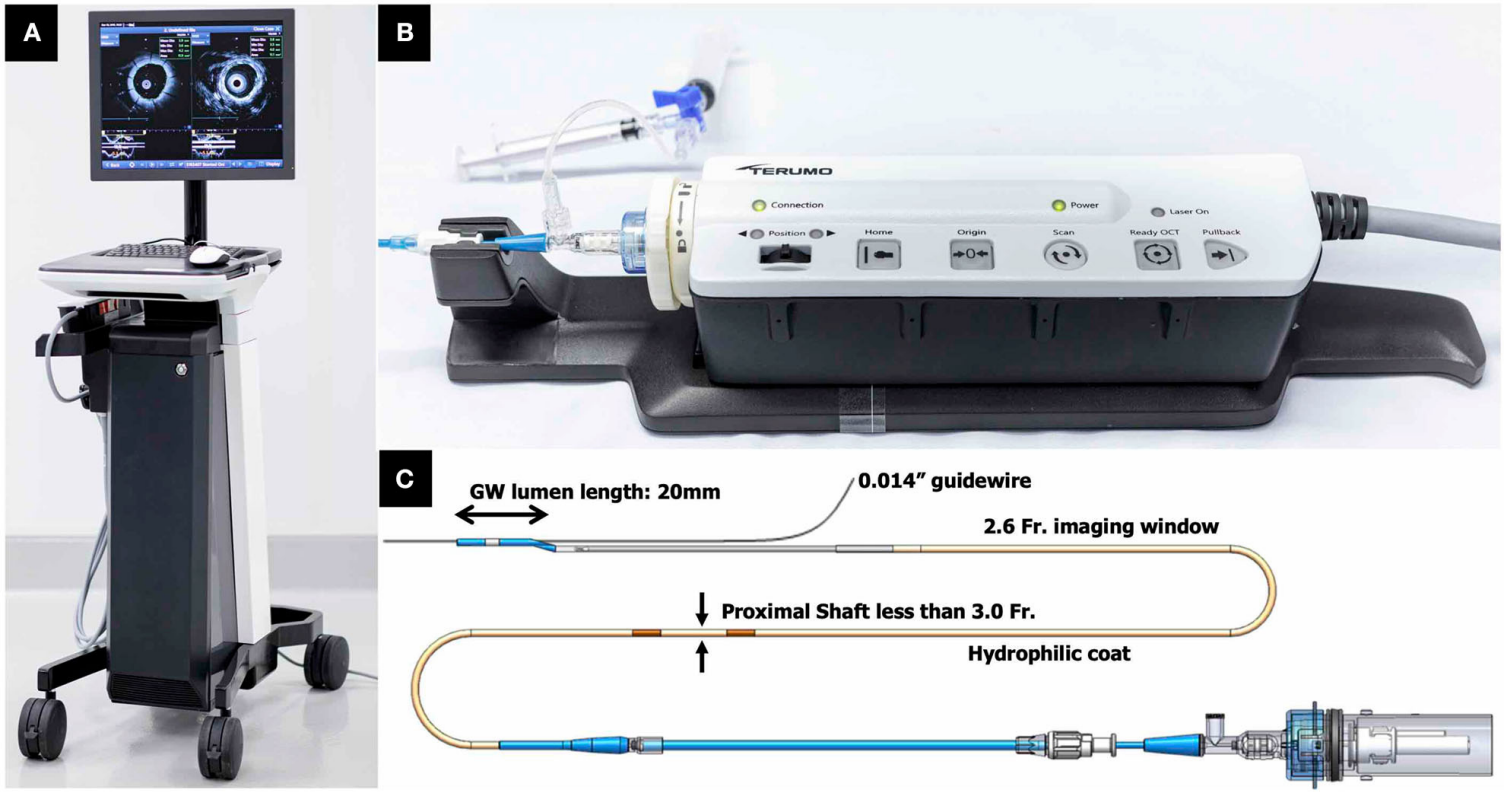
TERUMO hybrid IVUS–OCT catheter system; external appearances. (A) A whole appearance of system body. (B) Interface module. (C) Catheter technical specifications. IVUS, intravascular ultrasound; OCT, optical coherence tomography.
